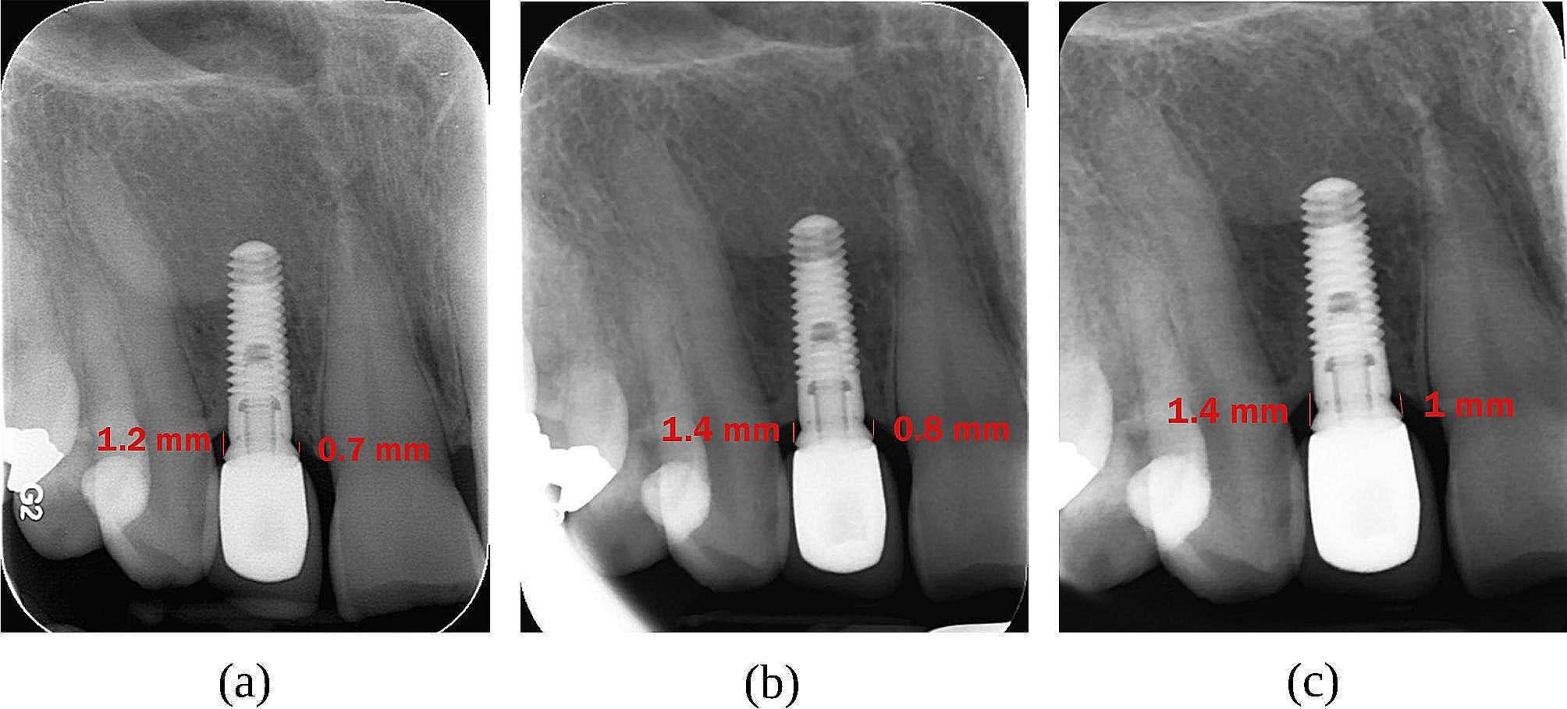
Radiographic assessments. (a) At the time of restoration delivery (baseline); (b) At six months follow-up; (c) At 12 months follow-up
